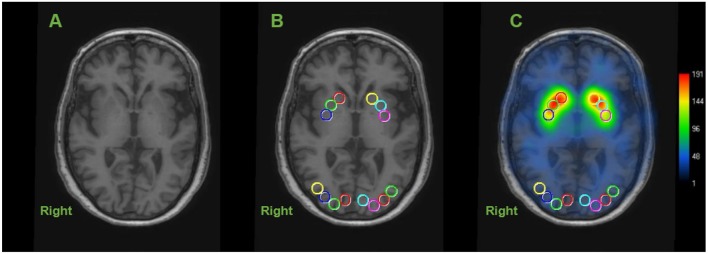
Representative image of regions of interest (ROIs) in dopamine transporter (DAT) image. Circular ROIs were manually placed 10 mm in diameter, in each hemisphere on the structural MRI for the caudate nucleus (CN), anterior putamen (AP) and posterior putamen (PP) and 8 on the occipital cortex as the reference region. Calculating each specific activity with DAT binding is estimated by the striatal-to-occipital ratio, defined as (striatum—occipital)/occipital counts. (A) Axial section of structural MRI (3-dimensional T1 weight image). (B) ROIs placed on an axial slice of MRI. (C) [^123^I]FP-CIT SPECT image overlaid onto the coregistered MRI.
